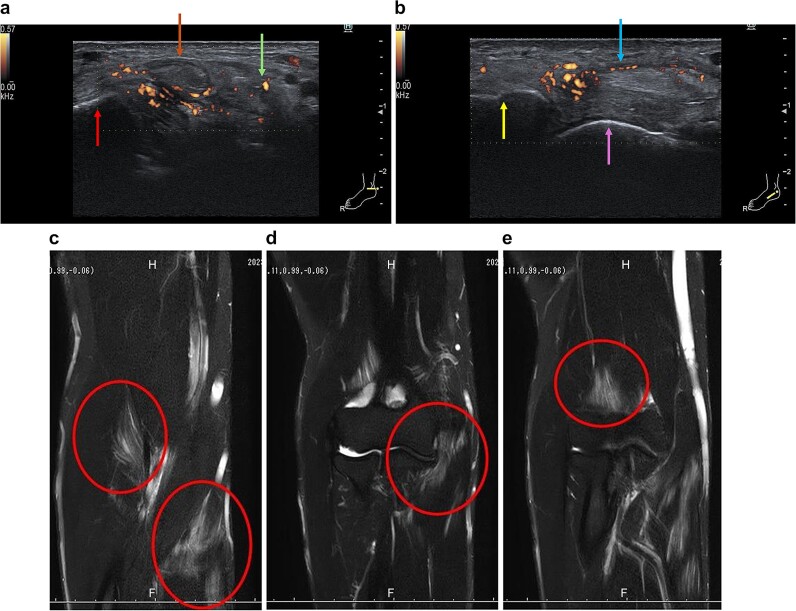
Ultrasound findings of the right ankle joint and magnetic resonance imaging findings of the right elbow at the time of referral to our hospital. a) Signs of tenosynovitis are observed in the posterior tibial tendon and flexor digitorum longus tendon (red arrow: Medial malleolus; brown arrow: Posterior tibial tendon; green arrow: Flexor digitorum longus tendon). b) There were signs of inflammation at the site of insertion of the posterior tibial tendon on the navicular tuberosity (yellow arrow: Navicular tuberosity; purple arrow: Talus; blue arrow: Posterior tibial tendon). c-e) Spectral Attenuated Inversion Recovery (SPAIR) sequence showing high signal intensity in multiple muscles around the right elbow.
